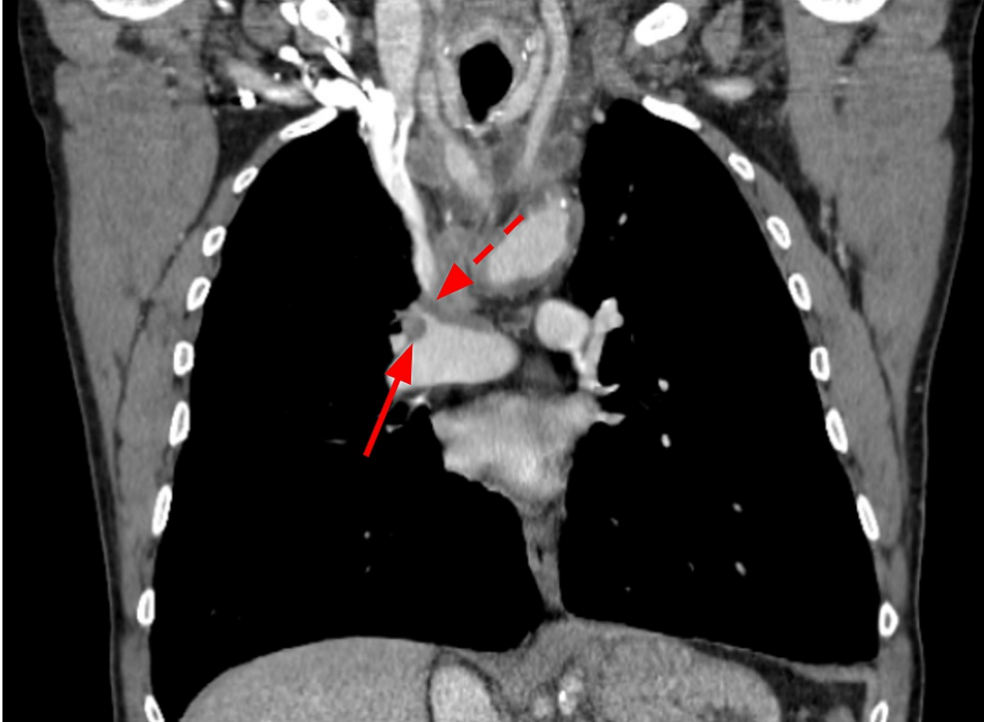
Computed tomography chest showing attenuation of the right pulmonary artery (arrow) and superior vena cava (dotted arrow).
